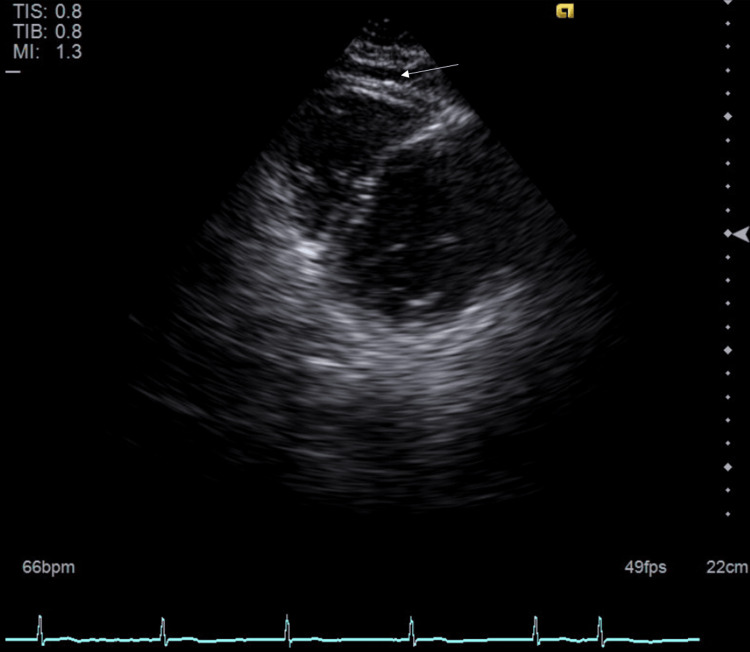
Transthoracic echocardiogram revealing preserved ejection fraction of 55%-60% and a small pericardial effusion (white arrow).
